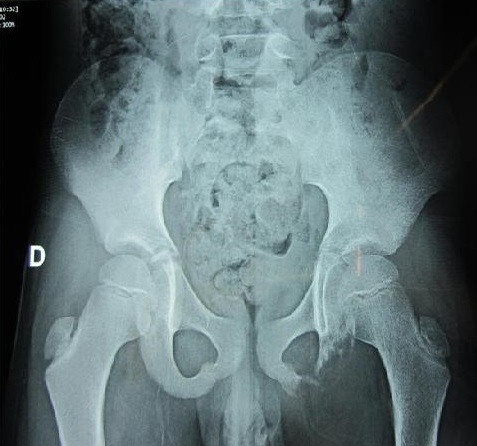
Radiographie du bassin montrant une lésion ostéolytique de l'ischion gauche
